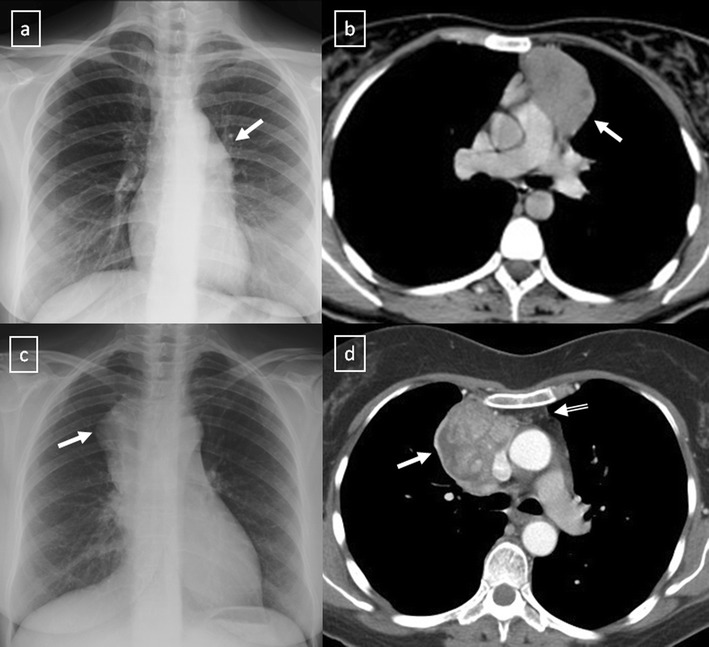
a, b Stage II thymoma (WHO type B1) in a 33-year-old woman who presented with myasthenia gravis. Frontal chest radiograph shows a hilum overlay sign (*arrow*) of a suggestive anterior mediastinal mass. Contrast-enhanced CT scan confirms the presence of a low-heterogeneous anterior mediastinal mass (*arrow*). Note the indentation of the arterial trunk pulmonary by the mass. c, d Stage III thymoma (WHO type B2) in a 54-year-old woman. Frontal chest radiograph reveals a lobulated mediastinal mass (*arrow*) on the right side. Contrast-enhanced CT scan demonstrates an enhanced anterior mediastinal mass (*arrow*) with infiltration of surrounding fat (*open arrow*)
